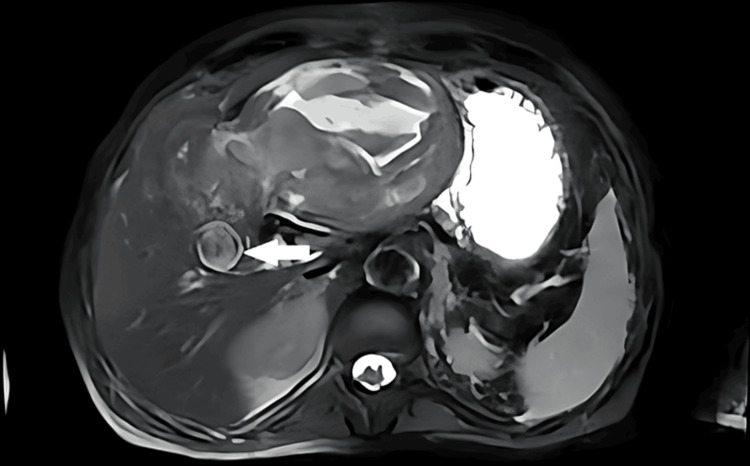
Magnetic resonance imaging showing pseudoaneurysm of the right hepatic artery (arrow) with large hematoma.
